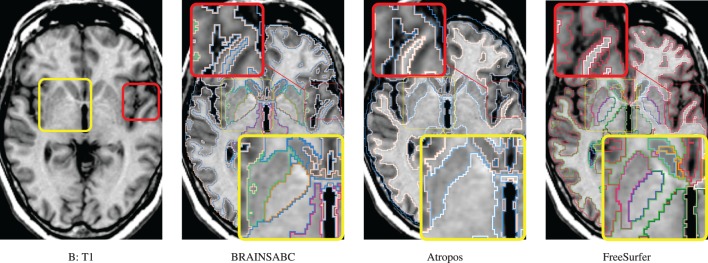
Visual Comparison of Scan B in Table 2. Tissue classification results from three applications, BRAINSABC, Atropos of ANTS Tools, and FreeSurfer on top of T1-weighted images, uni-modal processing. This subject present relatively small tissue ratio, reflecting brain atrophy progression to some extent. Again, Atropos were more parsimonious in the subcortical (yellow box) GM identification than others. Red box also underlines differences of tissue classifications on the cortical area. Note that without T2 modality, CSF classification results of BRAINSABC were more agreeable to the other two.
